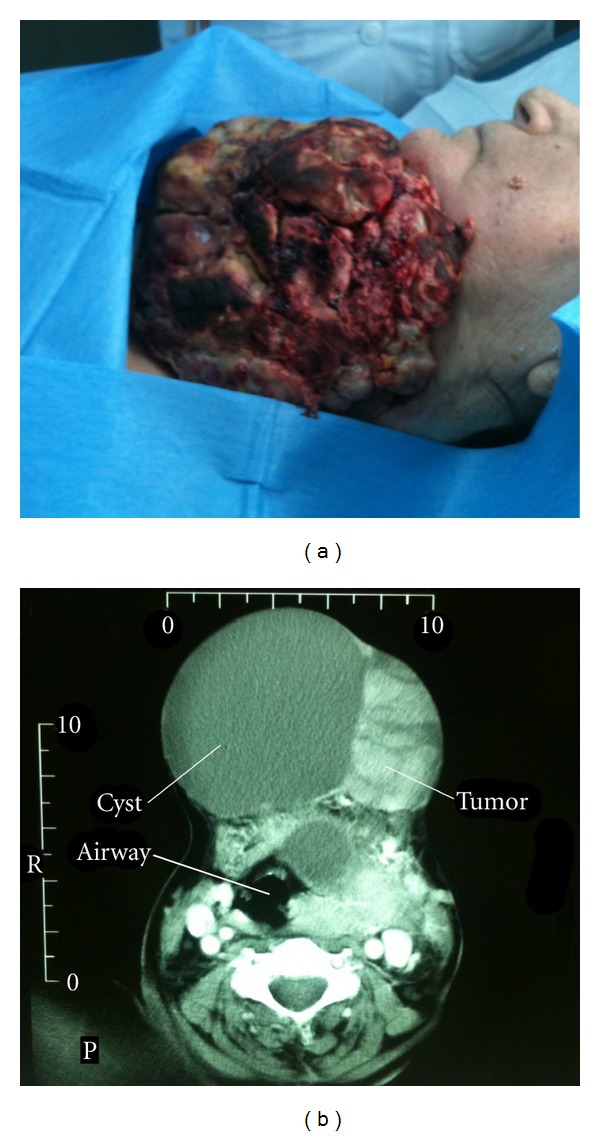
(a) Macroscopic appearance of the left hemorrhagic cauliflower-like side of the goiter. (b) Contrast-enhanced axial CT imaging shows a huge heterogenous mass localized to the left thyroid lobe and extending to the anterior neck space with a solid enhanced component and cystic areas. The trachea is displaced to the right side, and the mass reached the mediastinum.
